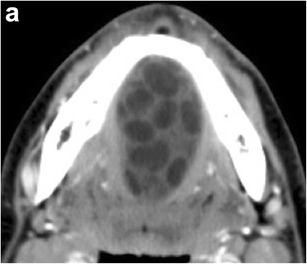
Dermoid cyst. Axial contrast-enhanced CT image shows a lesion with globules of fat (“sac of marbles”) in a fluid medium splaying the genioglossus muscles laterally to their respective ipsilateral sides
